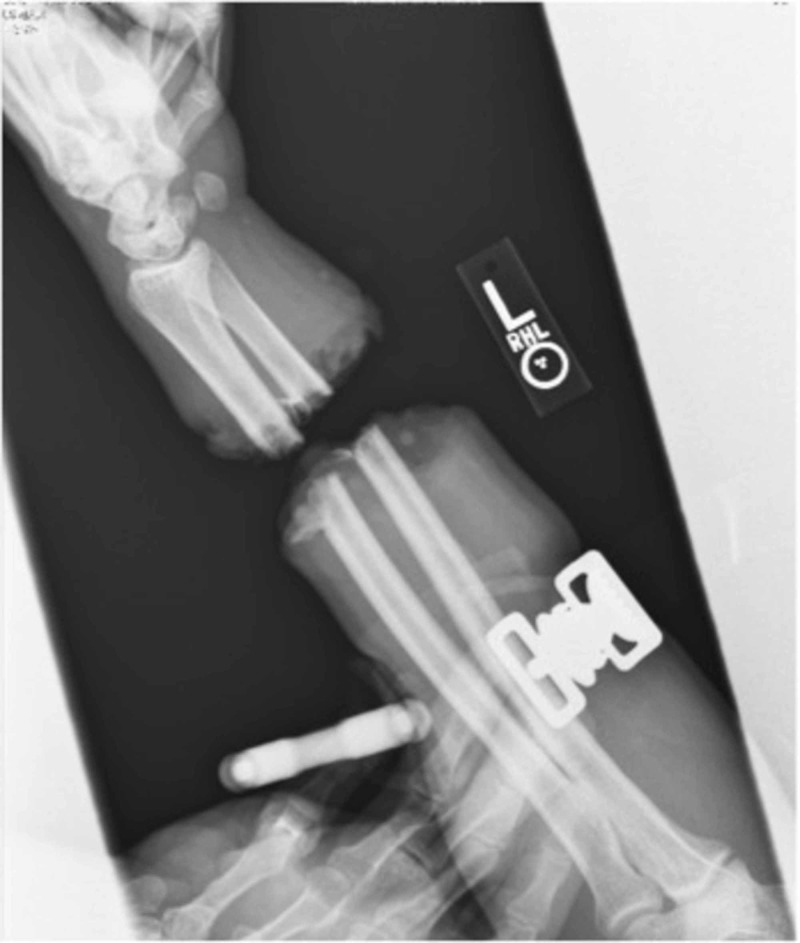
Forearm X-rays Forearm X-rays demonstrating a transverse radius and ulna forearm fracture at the mid-forearm location. X-rays demonstrate little comminution at the fracture sites.
